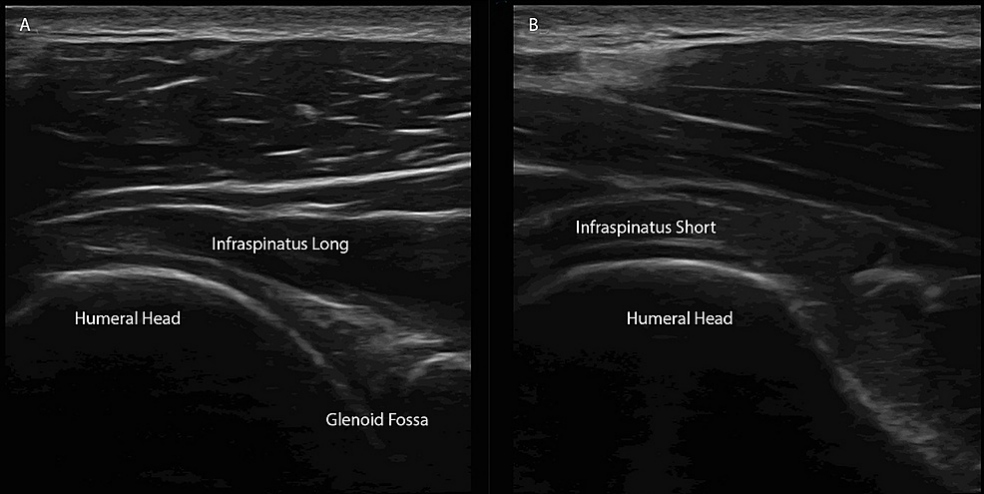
Infraspinatus (A) In this posterior view at the level of the glenohumeral joint beneath the scapular spine, the infraspinatus tendon may be visualized traversing above the glenohumeral joint. (B) The short view of the infraspinatus tendon is visualized with the ultrasound probe in the longitudinal orientation.
